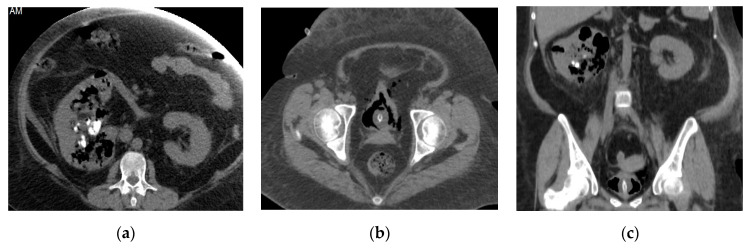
Emphysematous pyelonephritis and cystitis. Axial non-contrast CT images of the abdomen (a), pelvis (b), and coronal reformatted non-contrast CT image (c) show right emphysematous pyelonephritis in the form of diffuse parenchymal enlargement and destruction, multiple bubbly air locules involving the renal parenchyma and extends to the pelvicalyceal system with perirenal inflammatory fat strands and thickened fascia. Emphysematous cystitis can also be detected in the form of intramural air, and air within the bladder lumen with minimal perivesical extension.
